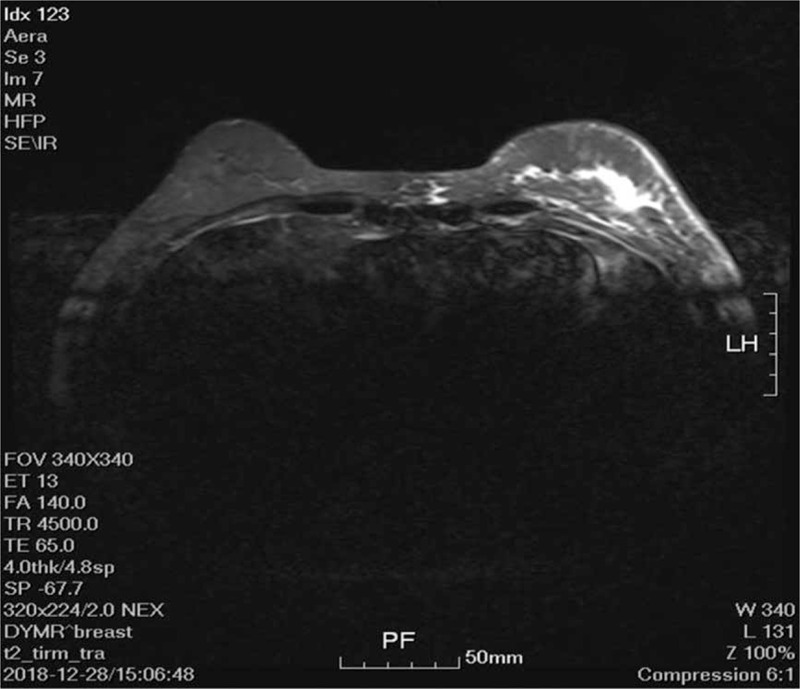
Nuclear magnetic resonance imaging (MRI): (1 axial MR images) results showed a left-breast mass patchy enhancement lesion and left axillary lymph node enlargement.
